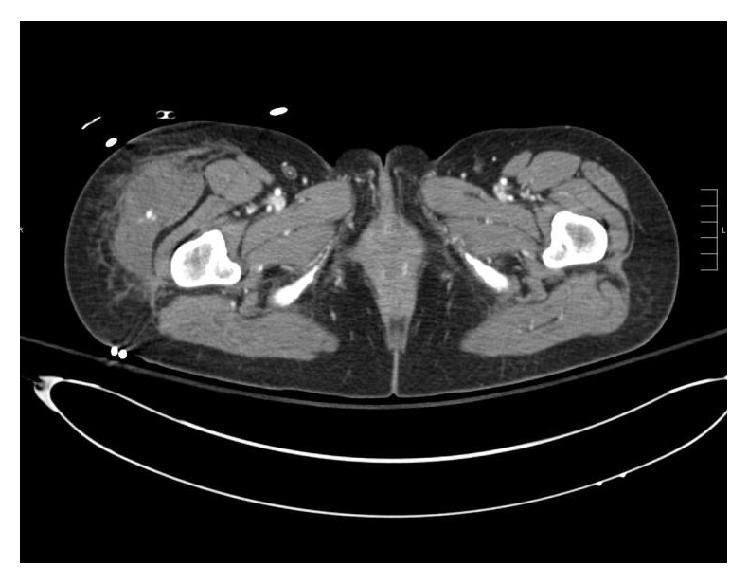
Contrast enhanced CT demonstrates an area of active extravasation and surrounding hematoma.
